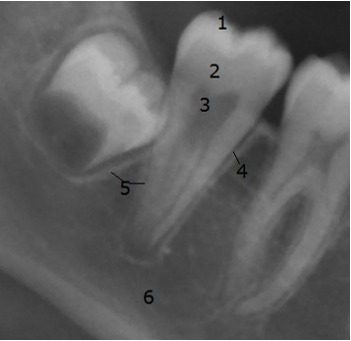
Cropped image of orthopantomogram showing lower molars of the right side. The third molar’s crown is radiologically of full radiopacity, the enamel to dentine border can be seen and roots are not developed: a continuous radiopaque line surrounds the developing tooth (1 = enamel, 2 = dentine, 3 = pulp cavity, 4 = periodontal space, 5 = lamina dura, 6 = cortical bone (lower border of mandible).
